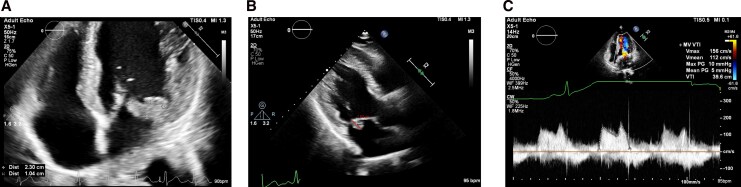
(*A*) Baseline transthoracic echocardiography showed posterior mitral valve leaflet vegetation 2.3 × 1.0 cm. (*B*) Transthoracic echocardiography after complete 11-week ceftriaxone showed persistent infective endocarditis over mitral valve > 1 cm. (*C*) Transthoracic echocardiography of post-mitral valve (MV) replacement showed MV prosthesis *in situ*, and there is no obvious leakage seen. The mean pressure gradient across MV prosthesis is 5 mmHg.
